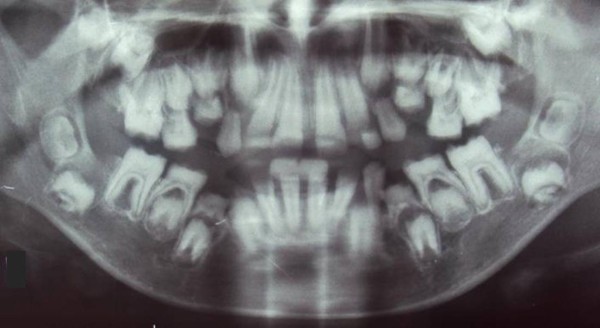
Panorex radiograph of the proband.
